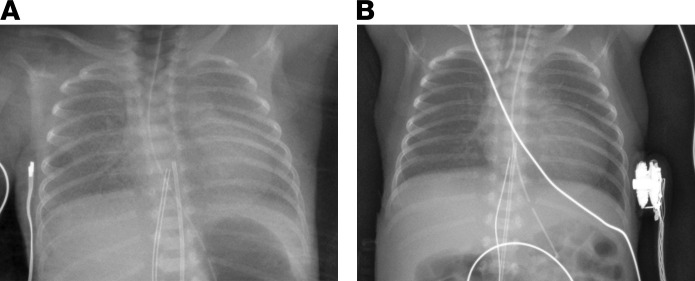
Chest radiographs of an intubated preterm infant with neonatal RDS exemplifies the benefits of surfactant treatment. (A) Before treatment, the baby was receiving CPAP with a fractional inspired oxygen concentration (FiO2) of 0.7. The classical radiographic findings showed hypoaerated lungs and air-filled bronchi, indicated by a light lung appearance with a fine granular pattern. (B) After intratracheal exogenous surfactant treatment, bilateral lung aeration improved with a reduction in FiO2 to 0.25. These improvements were reflected by darker lung appearance in the radiograph image. We thank Shoshana Newman-Lindsay and Francis Poulain at the University of California at Davis for providing these radiographic images, which were obtained with written informed consent.
